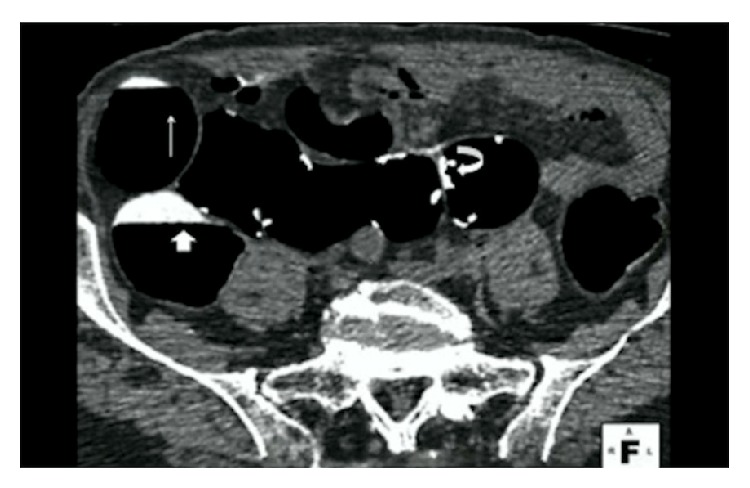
A 58-year-old man who applied 2-day dietary regimen. CT image in prone position shows tagged fluid (thin arrow) covering less than 25% of colonic lumen assigned to score 1 and 25–50% (thick arrow) assigned to score 2 for residual fluid in ascending colon. Some tagged feces (thin curved arrow) assigned to tag score 5 for residual solid stool, seen in sigmoid colon.
